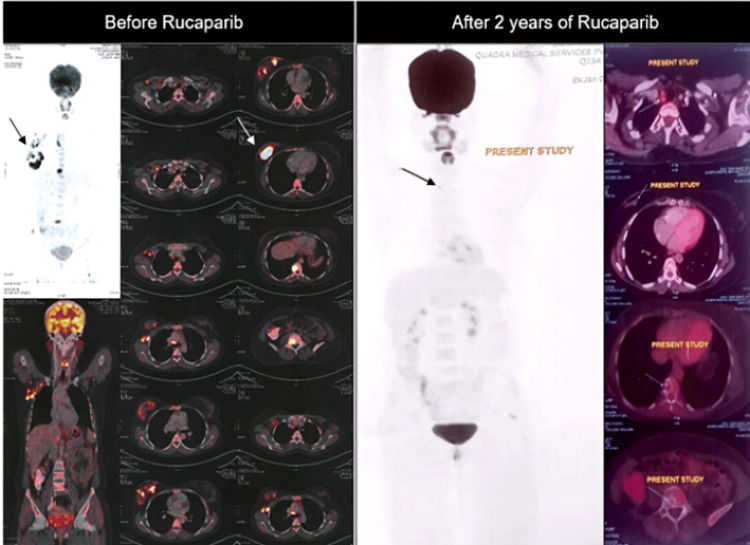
Case 2: sBRCA-Mutated MBC, Two Years Rucaparib Response on PET-CT Scan sBRCA, somatic breast cancer-mutated gene; MBC, metastatic breast cancer; PET-CT, positron emission tomography-computed tomography.
